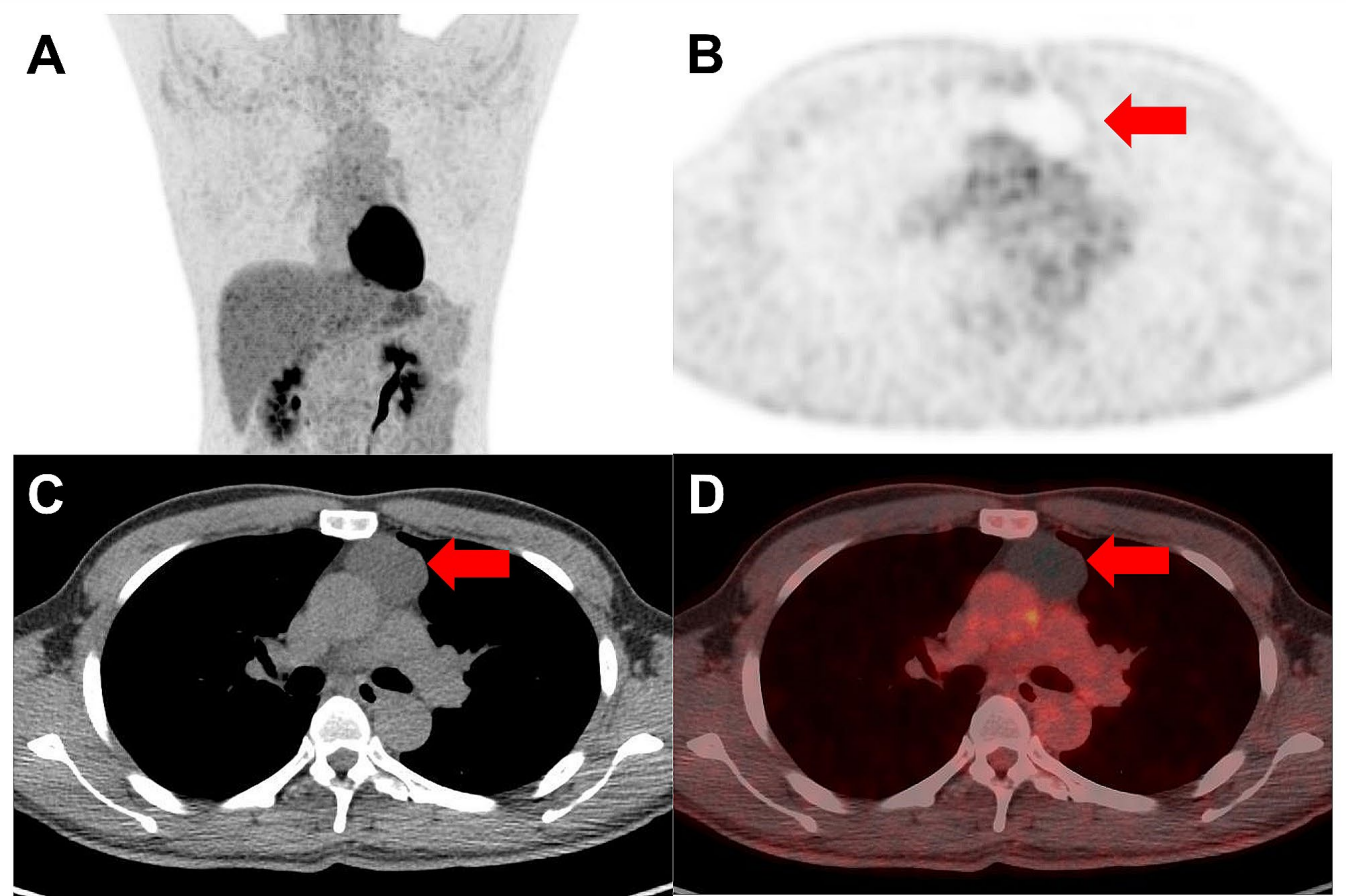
Representative [^18^F]FDG PET/CT from a 45-year-old man with a thymic cyst (red arrows). (A) Maximal intensity projection and (B) axial PET images show an anterior mediastinal mass with grade 1 uptake, central metabolic defect, and SUVmax of 1.0. (C) Axial CT demonstrates an anterior mediastinal mass with an oval shape, diameter of 4.4 cm, and attenuation of 12.5 HU. (D) Axial PET/CT fusion image
